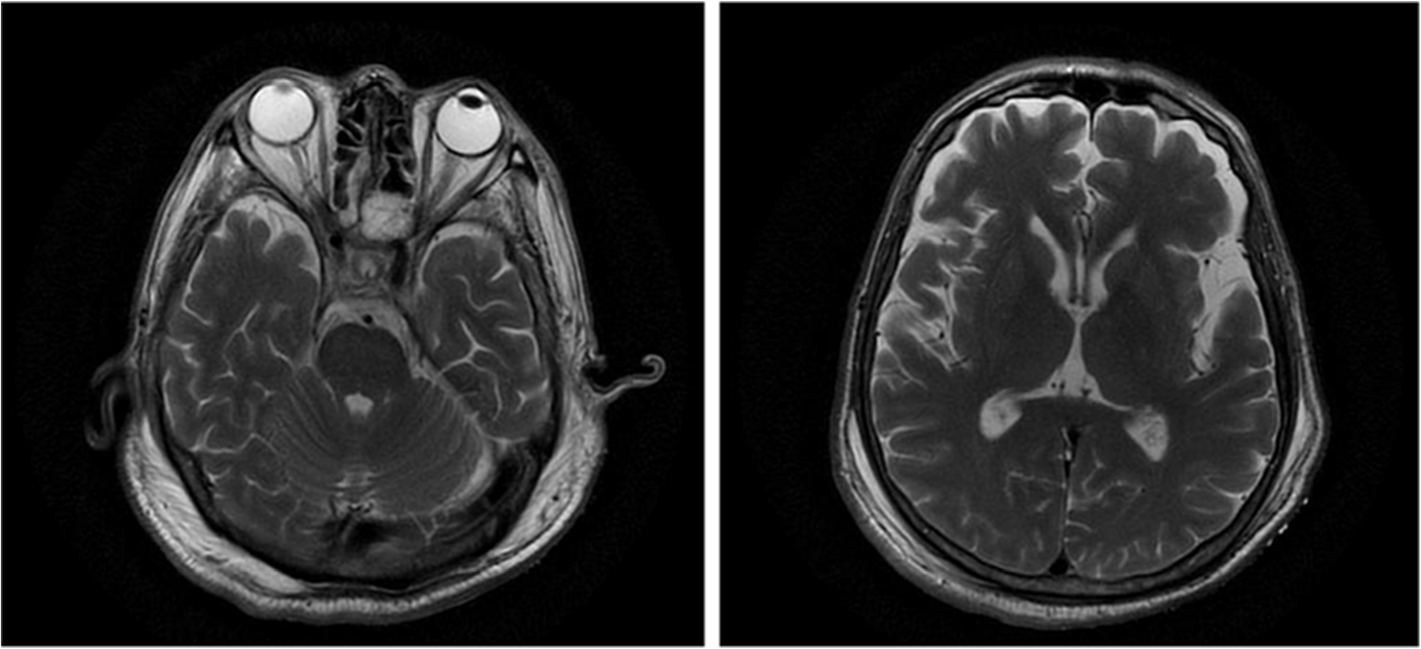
Brain MRI showing normal signal in mesocephalon (left) and basal ganglia region (right) in T2-weighted
